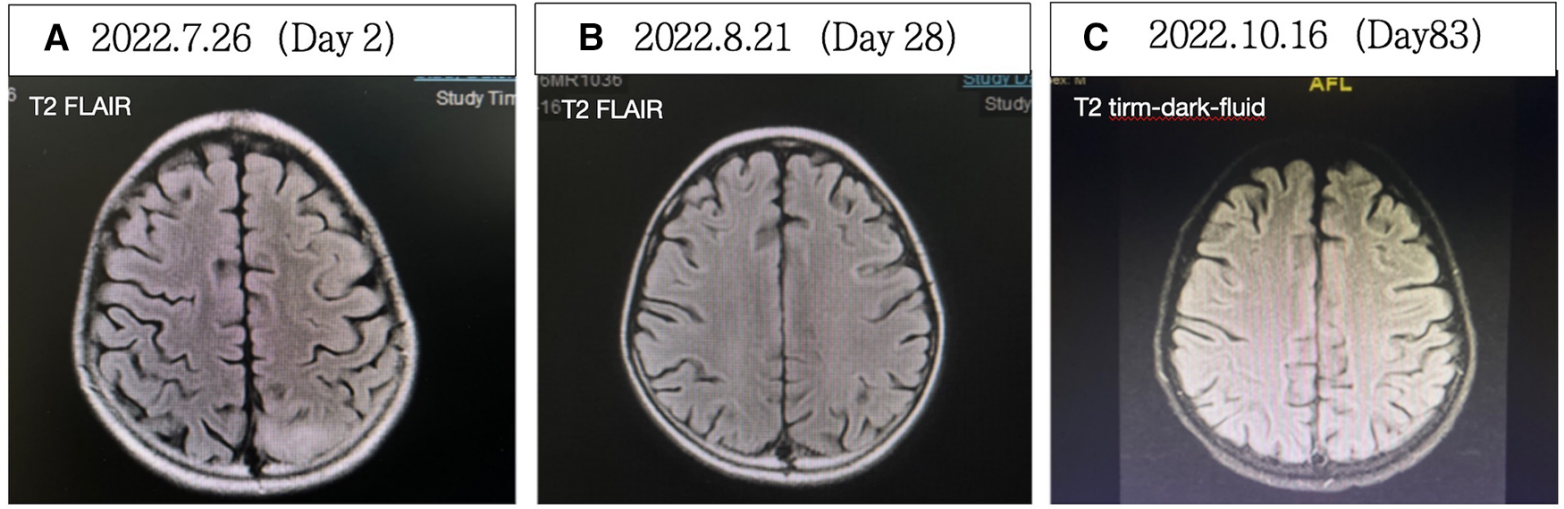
(A) Contrast-enhanced cerebral MRI showing abnormal signals in the left parieto-occipital lobe and thalamus on hospitalization day 2. (B) Cerebral MRI revealed less pronounced abnormal signals in the left parieto-occipital lobe and thalamus on hospitalization day 28. (C) No significant abnormal signals in the brain parenchyma were observed on hospitalization day 83.
